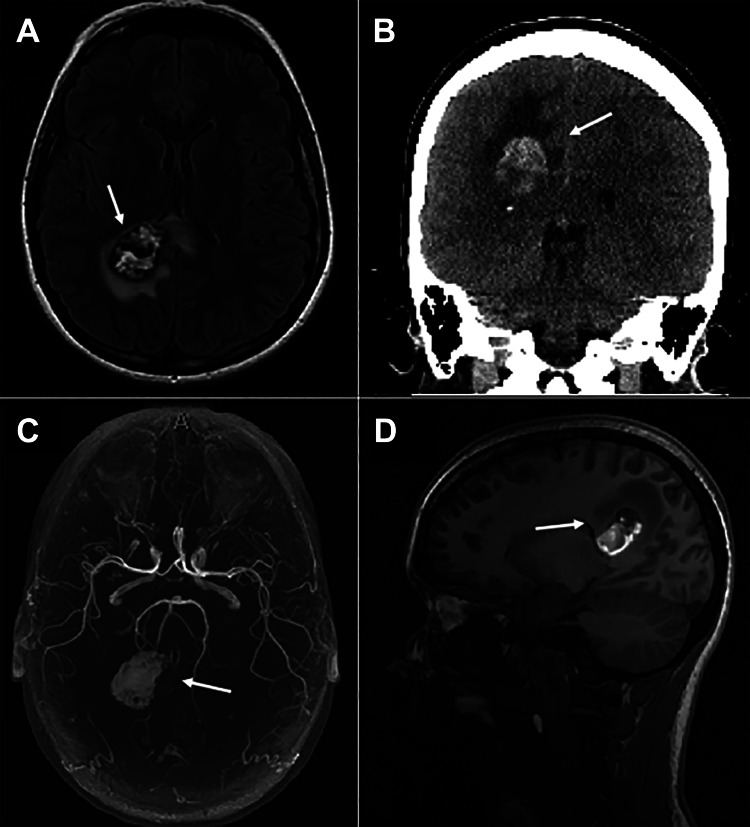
Magnetic resonance imaging of the enhancing mass. Preoperative (A) axial fluid-attenuated inversion recovery (FLAIR), (B) coronal CT, (C) axial 3D magnetic resonance angiogram, and (D) sagittal fast spoiled gradient echo (FSPGR) imaging obtained at presentation. The arrows in each image point to the large 3.0 × 2.5 cm hemorrhage near the occipital horn of the right lateral ventricle and the significant surrounding vasogenic edema.
